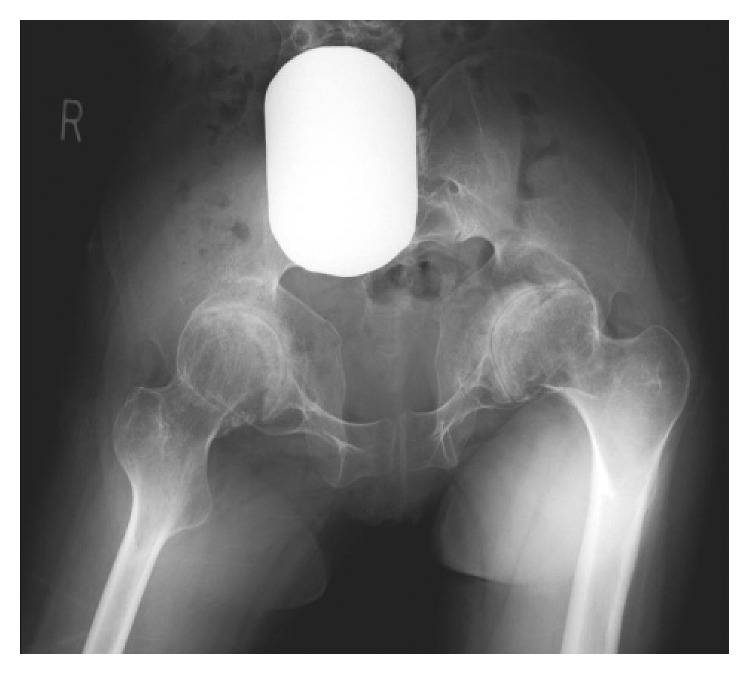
Preoperative radiograph showing end-stage osteoarthritis of the hip joints associated with large femoral heads.
